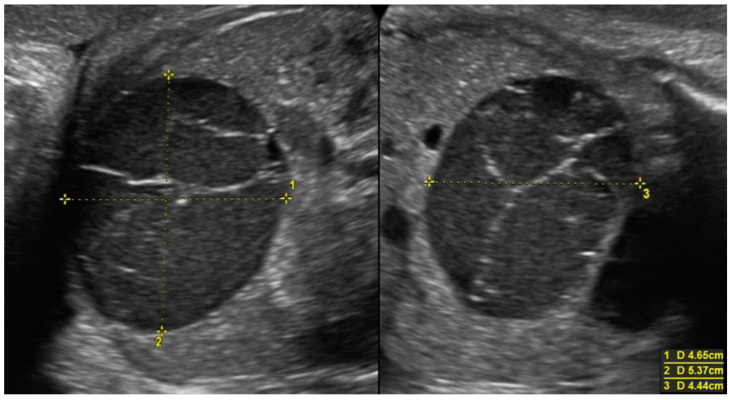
Voluminous ovarian cyst with hemorrhagic content in a female fetus at 35 weeks of pregnancy (46 × 54 × 44 mm). At 37 weeks of pregnancy, the formation regressed spontaneously, and at the ultrasound after delivery, multifollicular ovaries were described.
